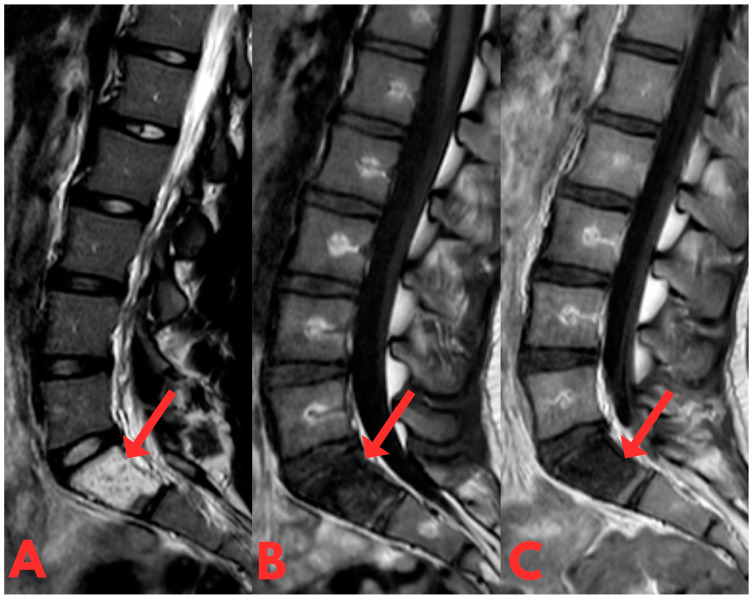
First T1- and T2-weighted sagittal contrast-enhanced MRI of the lumbar spine (T2W_TSE, T1W_TSE, T1W_TSE c+ sag) showing a sclerotic lesion with a hyperintense signal on T2 (A) and hypointense on T1 (B,C), which occupies practically the entire body of the S1 vertebra. Spread is not seen to the anterior epidural space or the prevertebral space.
